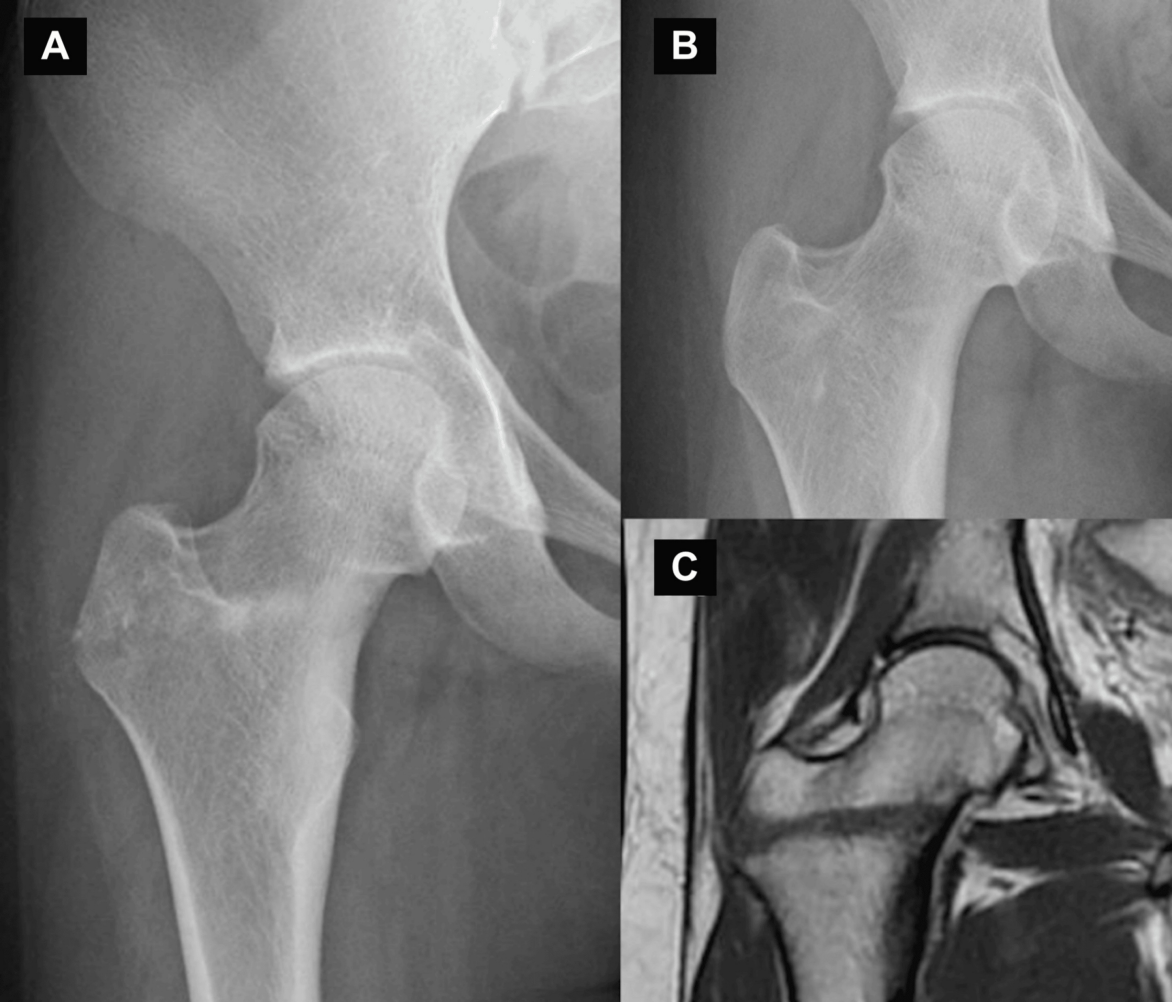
Postoperative images (A) Immediately after the operation; (B) nine months after the operation. The bony bump remains at the femoral head-neck junction. (C) T2-weighed magnetic resonance imaging shows the disappearance of the high signal intensity in the right hip joint.
